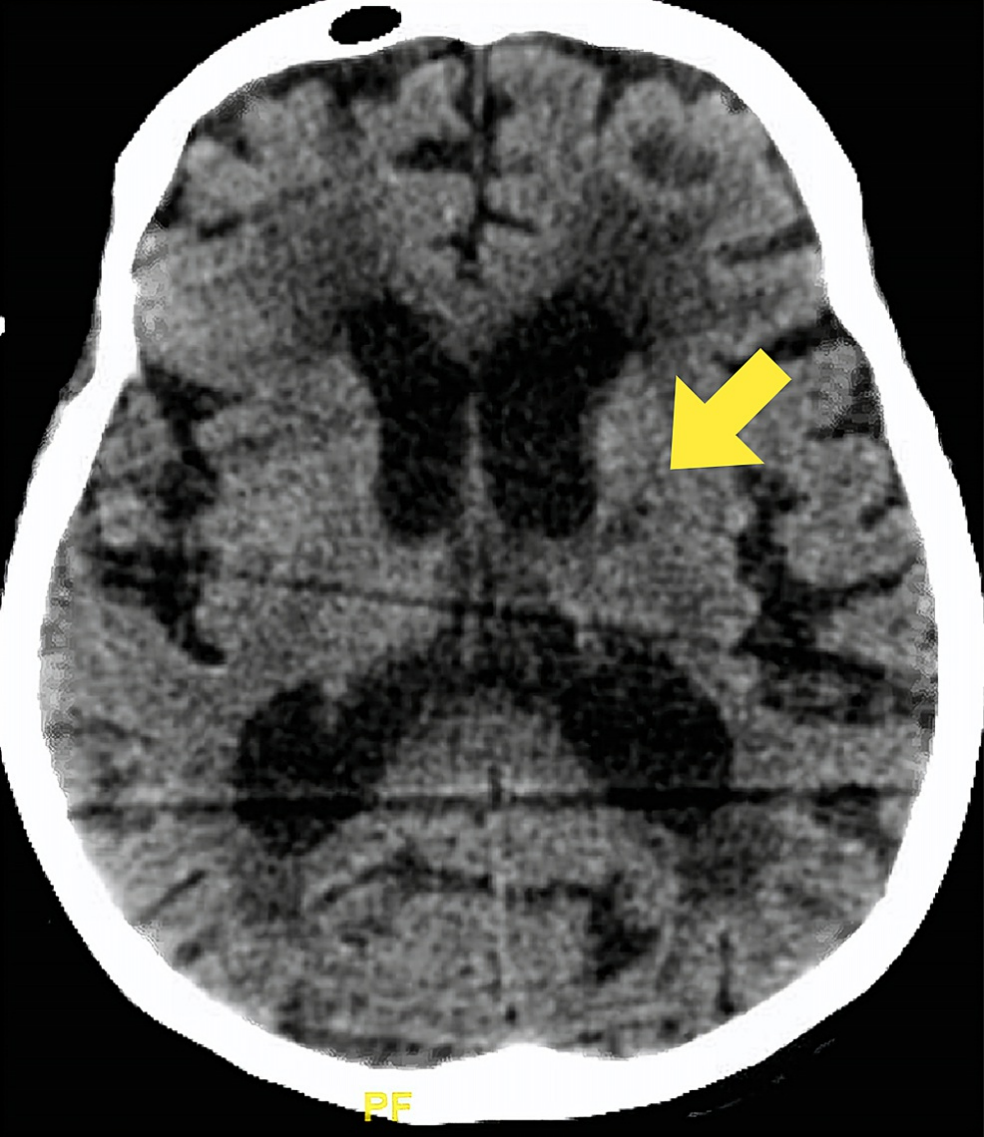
Computed tomography of the brain showing an infarction in the left basal ganglia and thalamus
